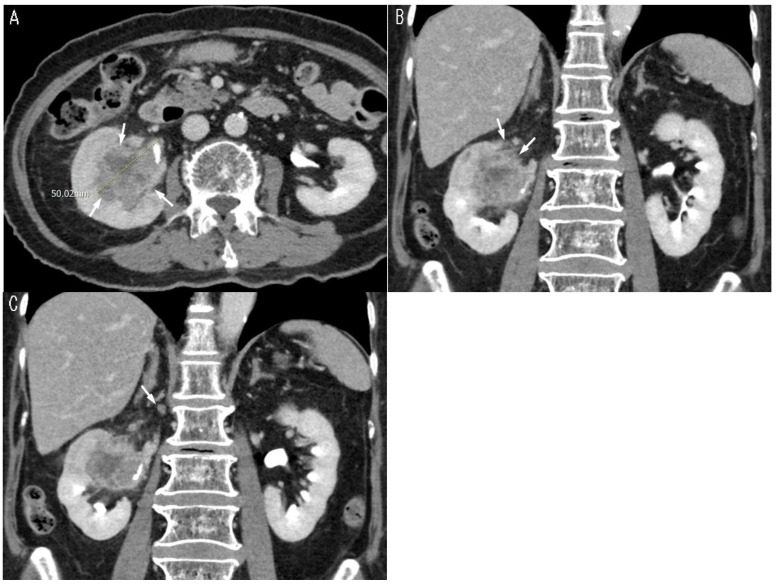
CT images of an 82-year-old female patient with renal pelvis urothelial carcinoma. (A) Axial preoperative CT image shows a partially ill-defined soft-tissue mass (arrows) in the right renal pelvis without pelvicalyceal dilatation. The mass measures 5 cm in maximum diameter and was confirmed as high-grade urothelial carcinoma. (B) Coronal CT image shows peritumoral fat infiltration (arrows) in the upper aspect of the soft tissue mass involving the right renal pelvis. (C) Coronal CT image shows a small right hilar lymph node (arrow), 4.5 mm in short-axis diameter, confirmed as metastatic following lymph node dissection.
